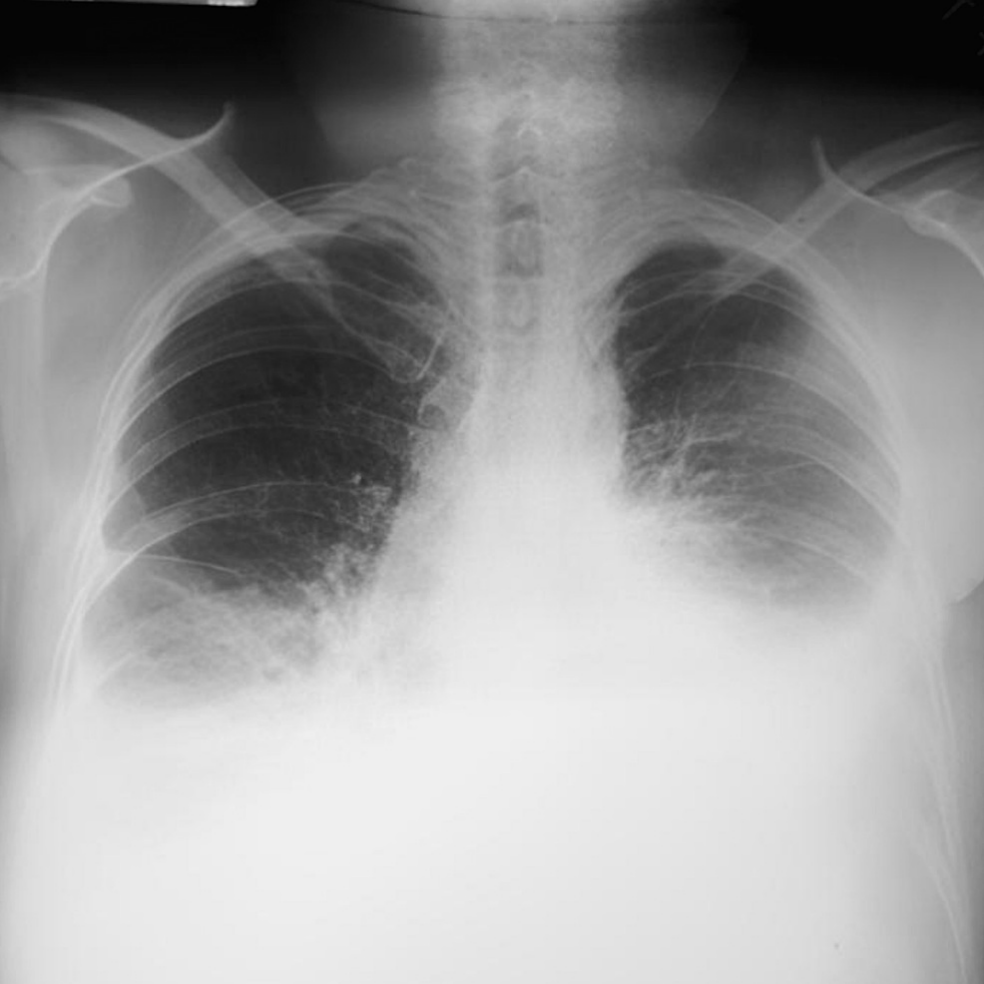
Chest x-ray PA view shows blunting of the bilateral costophrenic angle, suggestive of bilateral pleural effusion. PA: postero-anterior.
